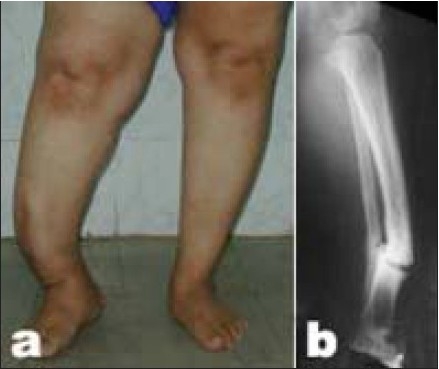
(a) Clinical photograph and (b) Radiograph (lateral view) of a female patient aged 51 years with leg deformity after being treated with interlocking nail for Type II open distal tibial fracture which shows hypertrophic nonunion after nail extraction following infection.
